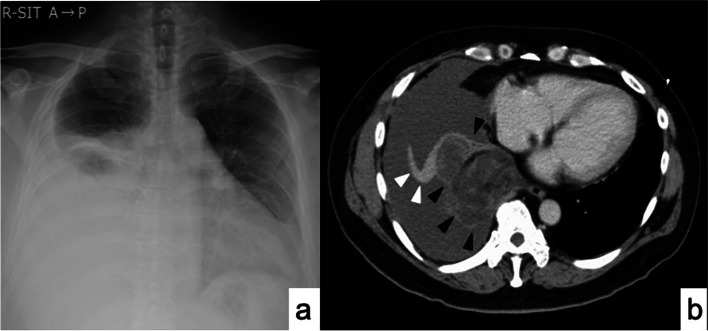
Chest x-ray showing a massive right pleural effusion. b. Enhanced chest CT showing a massive right hemothorax and a 78 × 70 mm mass in the right lower lung lobe (▲). The contrast agent (△) had leaked into the thoracic cavity around the tumor
